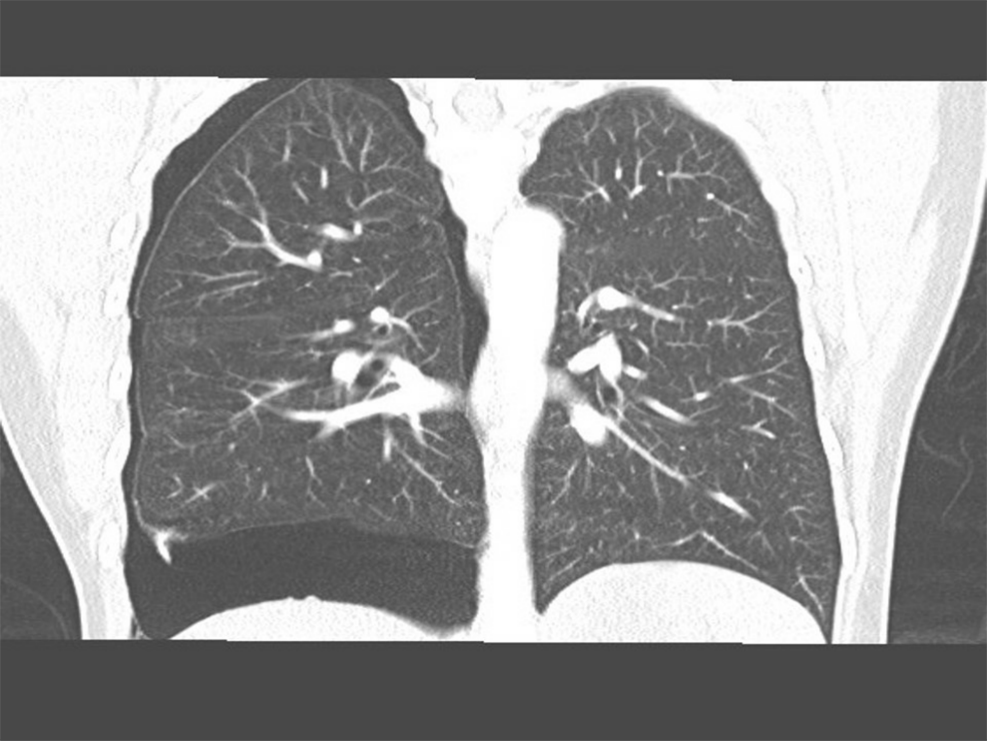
Coronal plane of computed tomography chest showing large right pneumothorax occupying approximately 20% of right hemithorax.
